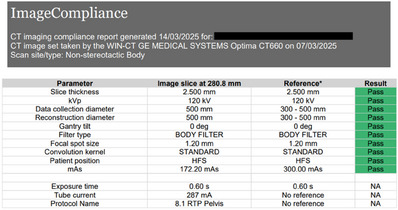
Sample CT QA report generated by ImageCompliance V2.0.
